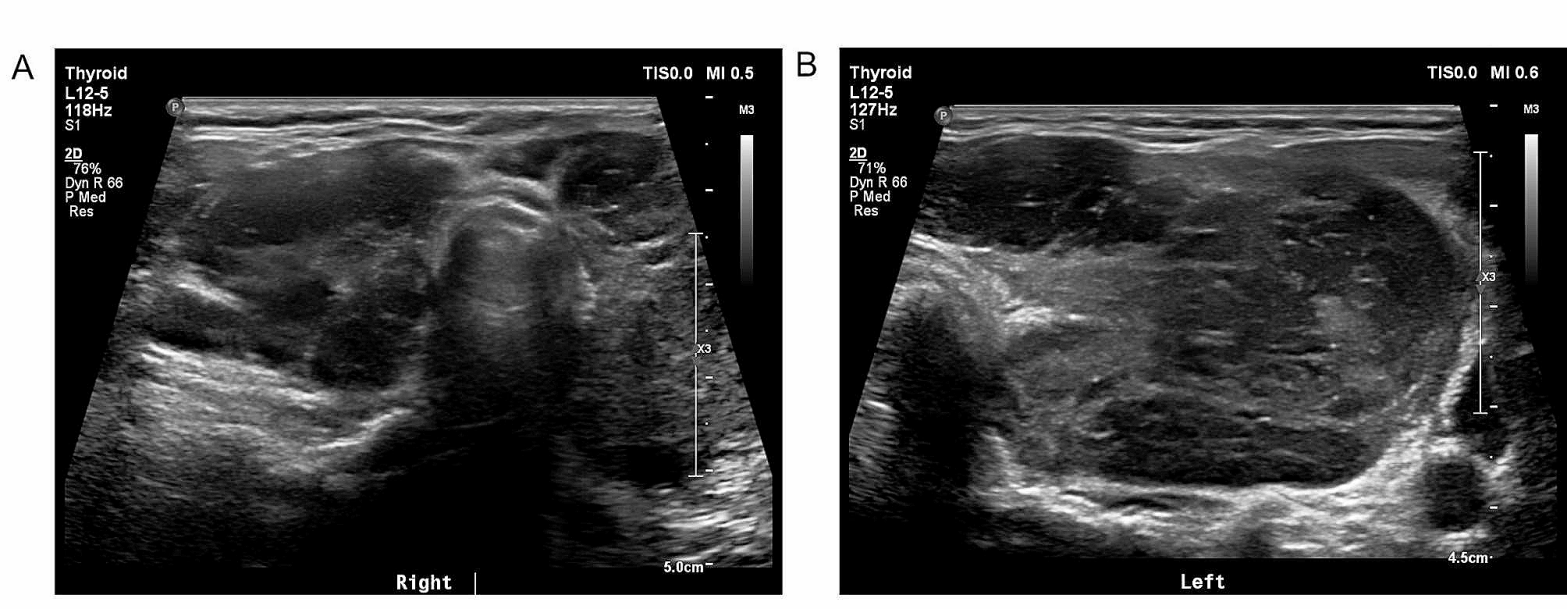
The transverse section of the thyroid from a type-A primary thyroid lymphoma patient. The anterior-posterior diameter was 2.7 cm for the right lobe (A), and 3.2 cm for the left lobe (B). In addition, sonographic features of ultra-hypoecho and strip echo were present for this patient
